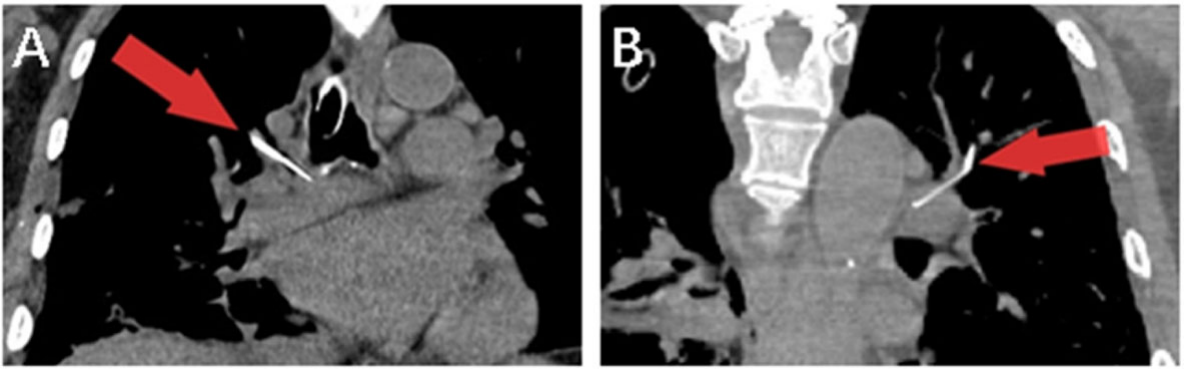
CT scans during the operatin. a In the first CT scan, the red arrow indicates the hook wire sliding into artery of apical segment of right upper lobe; b In the second CT scan, the red arrow indicates the localizer sliding to the artery of apicoposterior segment of left upper lobe. CT, computed tomography
